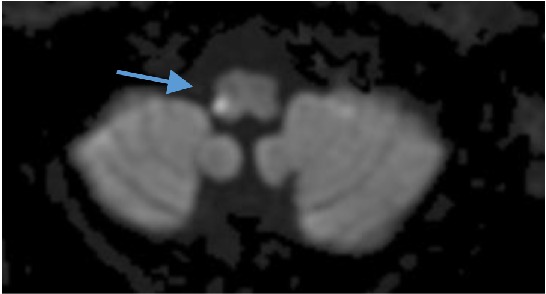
Diffusion weighted MRI demonstrating an area of restricted diffusion in the right posterior lateral medulla (arrow), consistent with an acute infarct.
